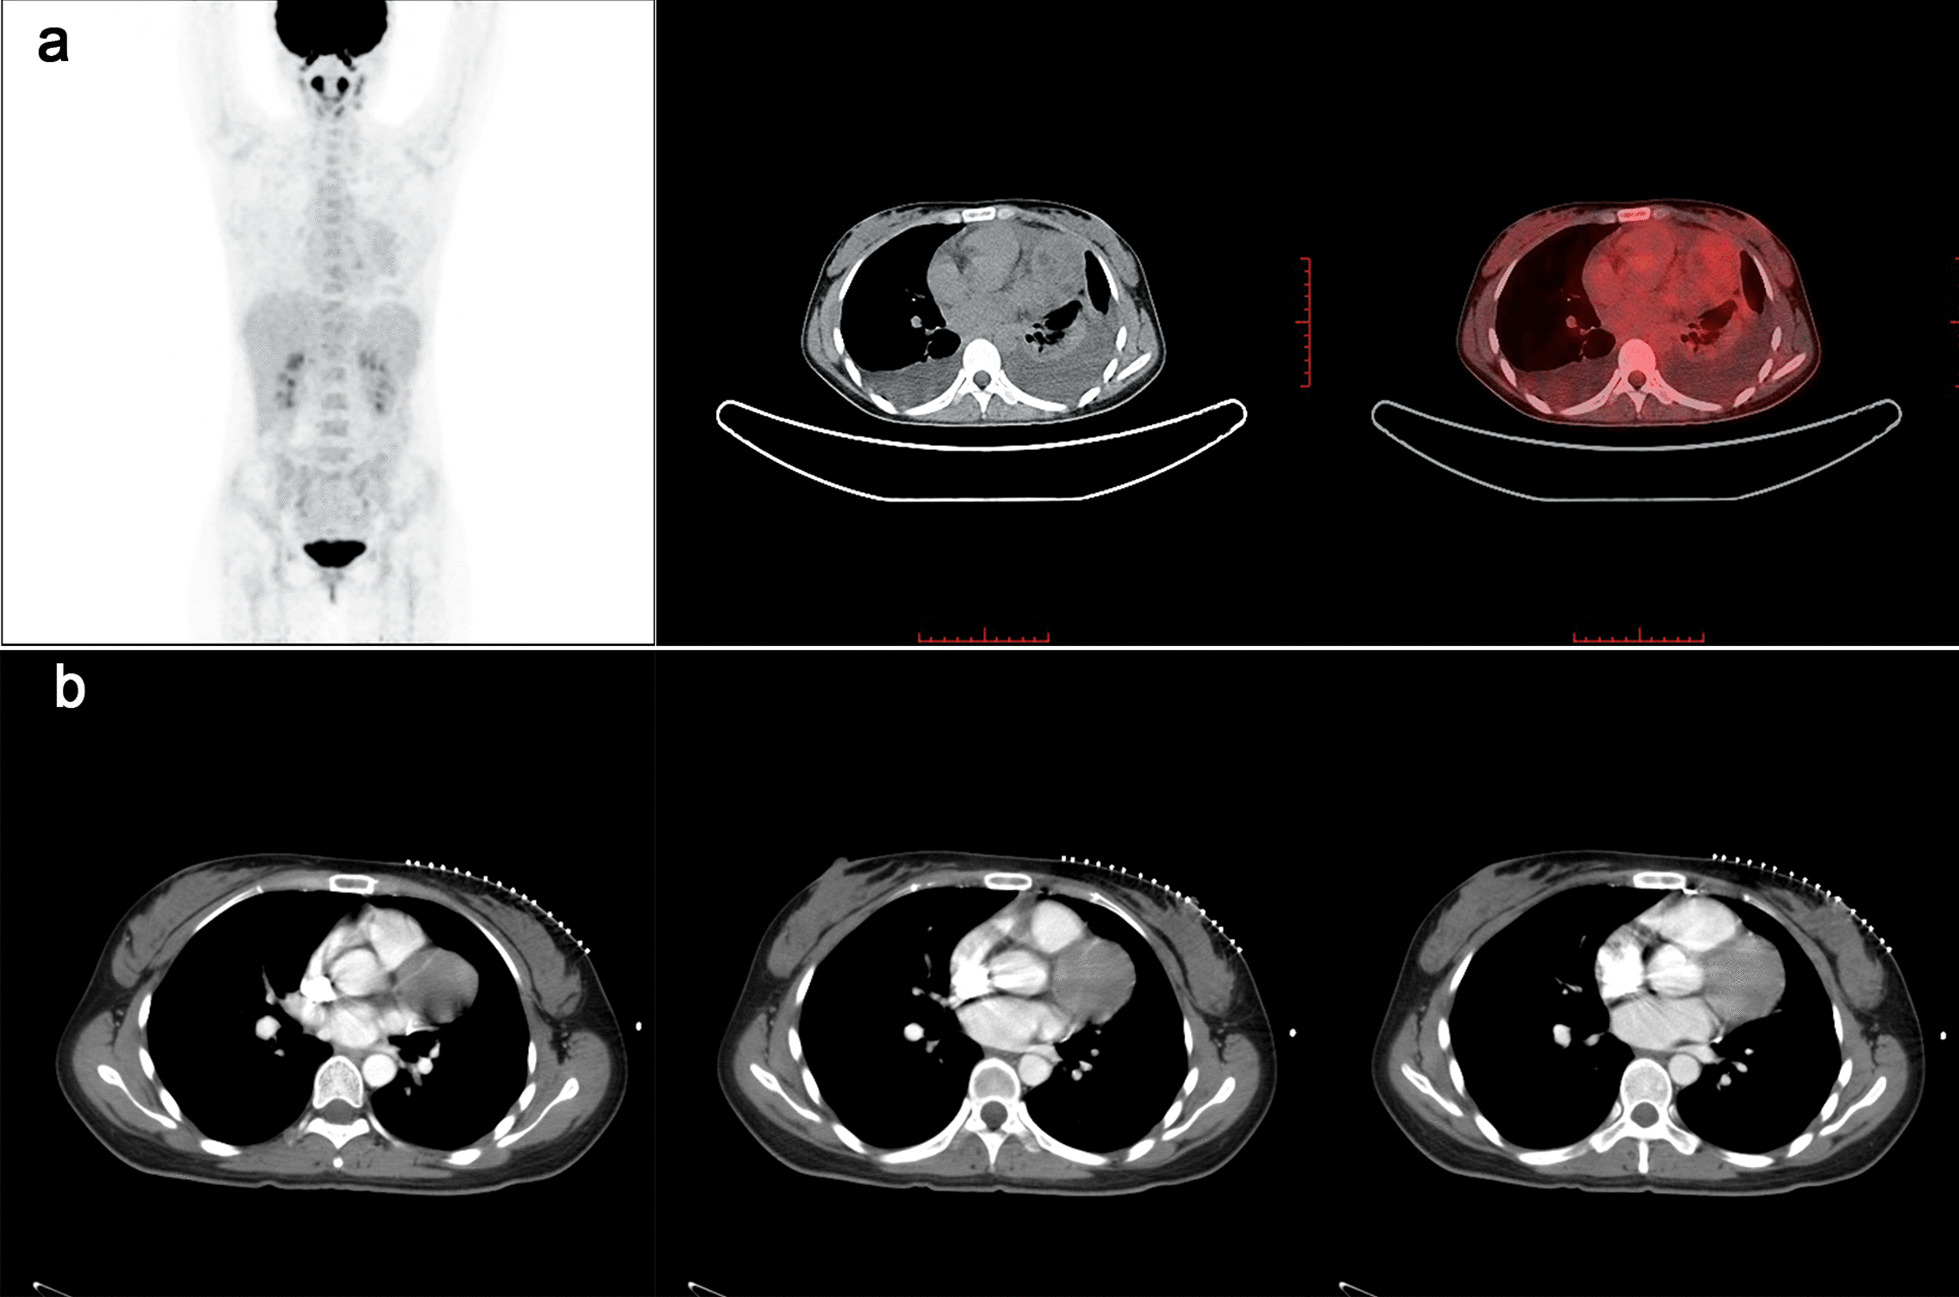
The imaging characteristics of the lesion before PNET excision. a Positron emission tomography-CT shows a soft tissue density with heterogeneous hypermetabolism. b Contrast-enhanced CT scan of the mediastinum, the mass in the left parietal pericardium showed no significant enhancements
